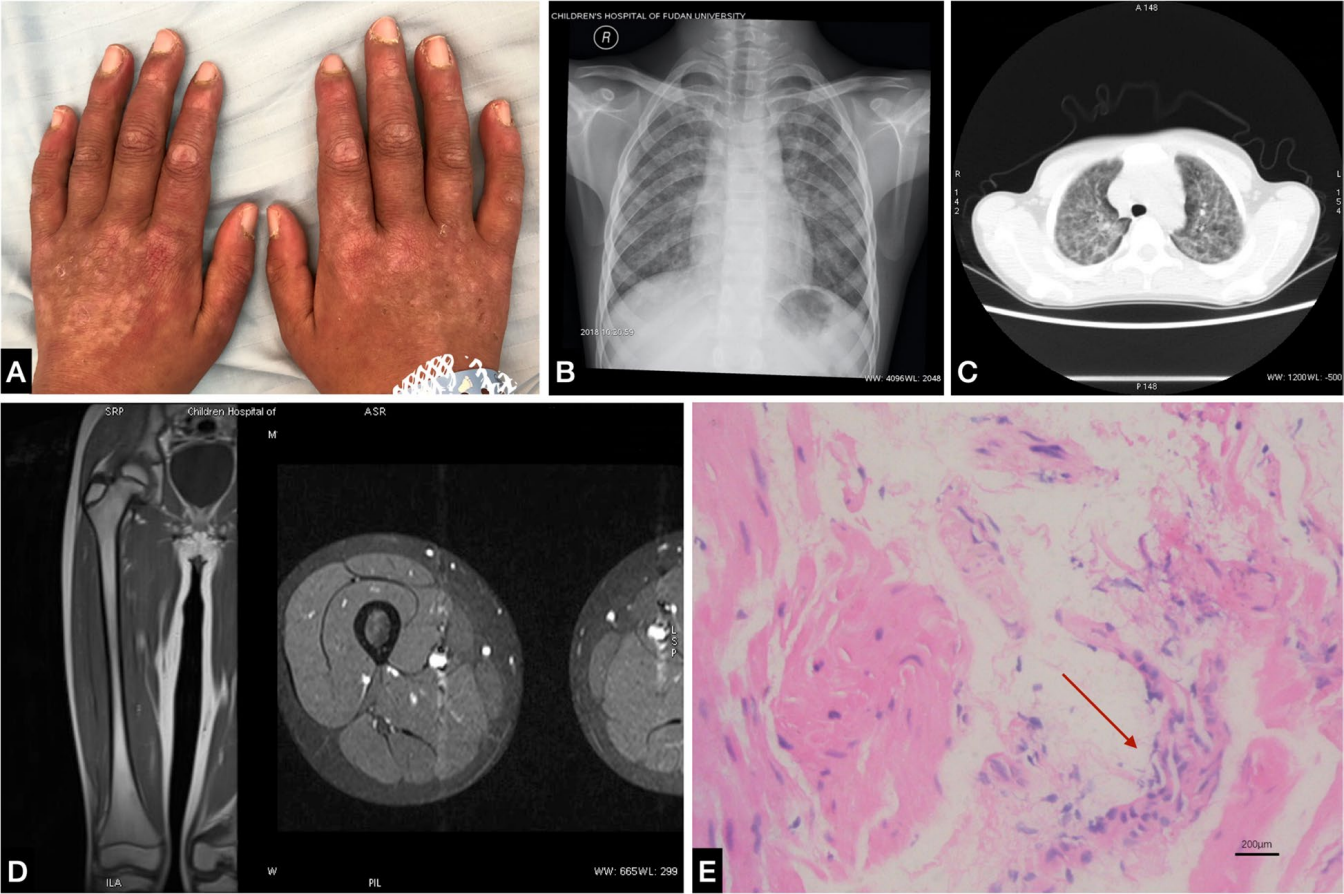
Gottron’s sign, radiological findings and pathological biopsy of CAJDM-ILD. A: Typical Gottron papules are present over the knuckles; B: Chest radiograph shows diffused interstitial infiltration in both lungs; C: Chest HRCT shows diffused interstitial lung changes as grid sign with fibrous streak shadow and right pleural reaction; D: Enhancement MRI of the thigh muscle shows no obvious abnormalities; E: Pathological biopsy of the basal ridge of the right lung under a bronchoscope shows chronic inflammation of the mucosa with epithelial cells and more neutrophil infiltration (Leica DM750, Leica Application Suite, 2560 × 1920 Full Frame HQ, original magnification× 200)
